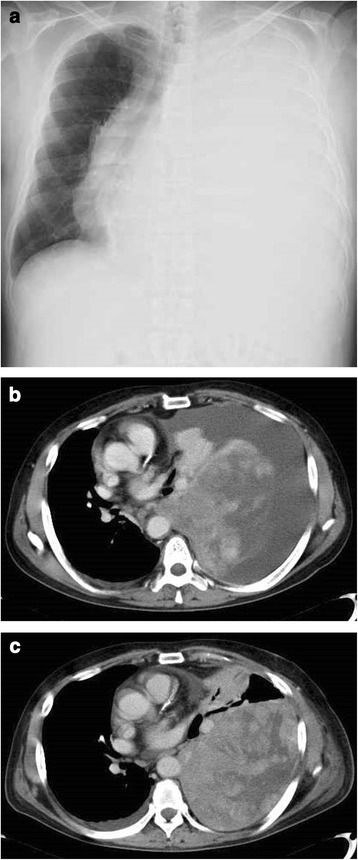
Preoperative imaging studies. The chest radiogram shows a large pleural effusion in the left thoracic cavity and a mediastinum shift to the right (a). Contrast-enhanced computed tomography (CT) on admission shows a 20-cm tumor and pleural effusion in the left thoracic cavity (b). Contrast-enhanced CT a month after admission shows that the tumor has rapidly grown and the right pleural effusion has increased since admission (c)
